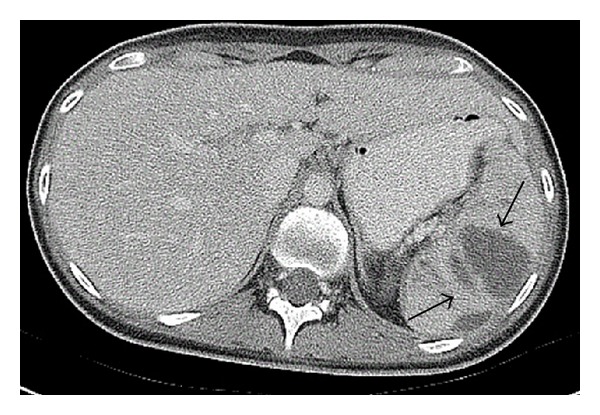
Splenic abscesses noted on CT scan (arrows).
